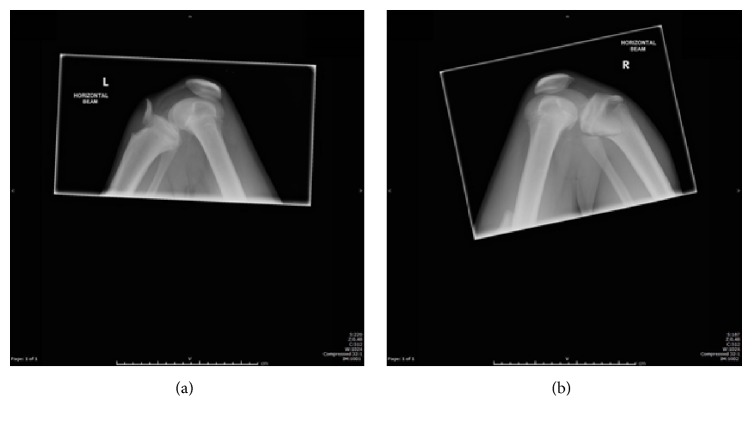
Plain radiographs of left (a) and right (b) proximal tibial avulsion fractures, respectively.
